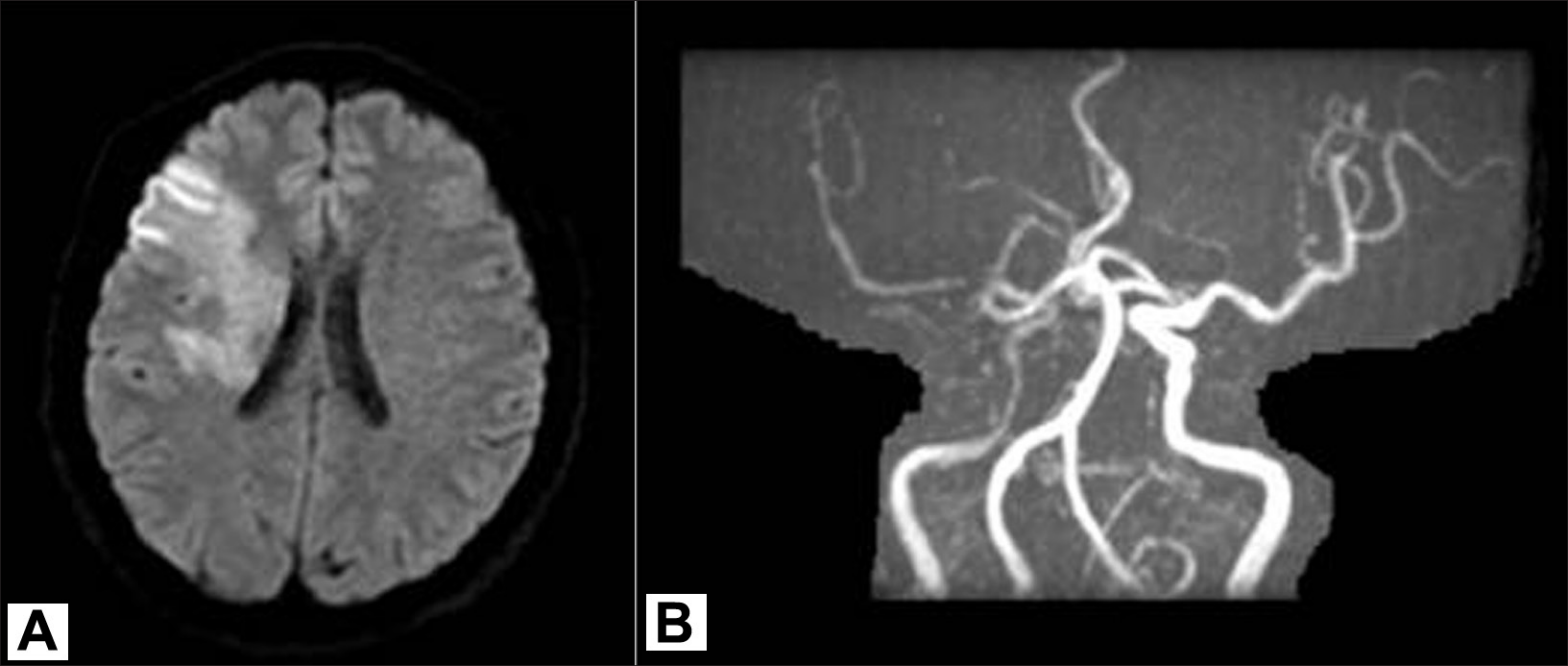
MRI and MRA scans showing acute infarction in the right frontotemporal area involving the right basal ganglia and corona radiata (A), and segmental narrowing of the siphon of the right ICA and the M1 segment of right MCA (B).
